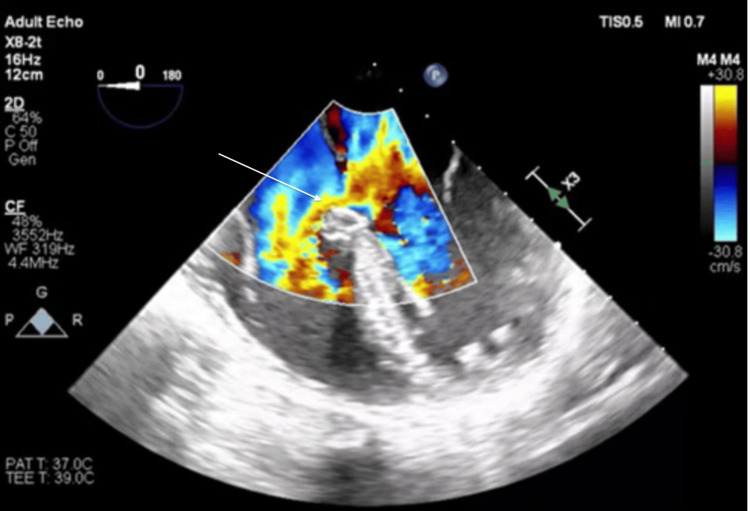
TEE mid-esophageal view with color Doppler showing the atrioventricular septal defect TEE, transesophageal echocardiography
